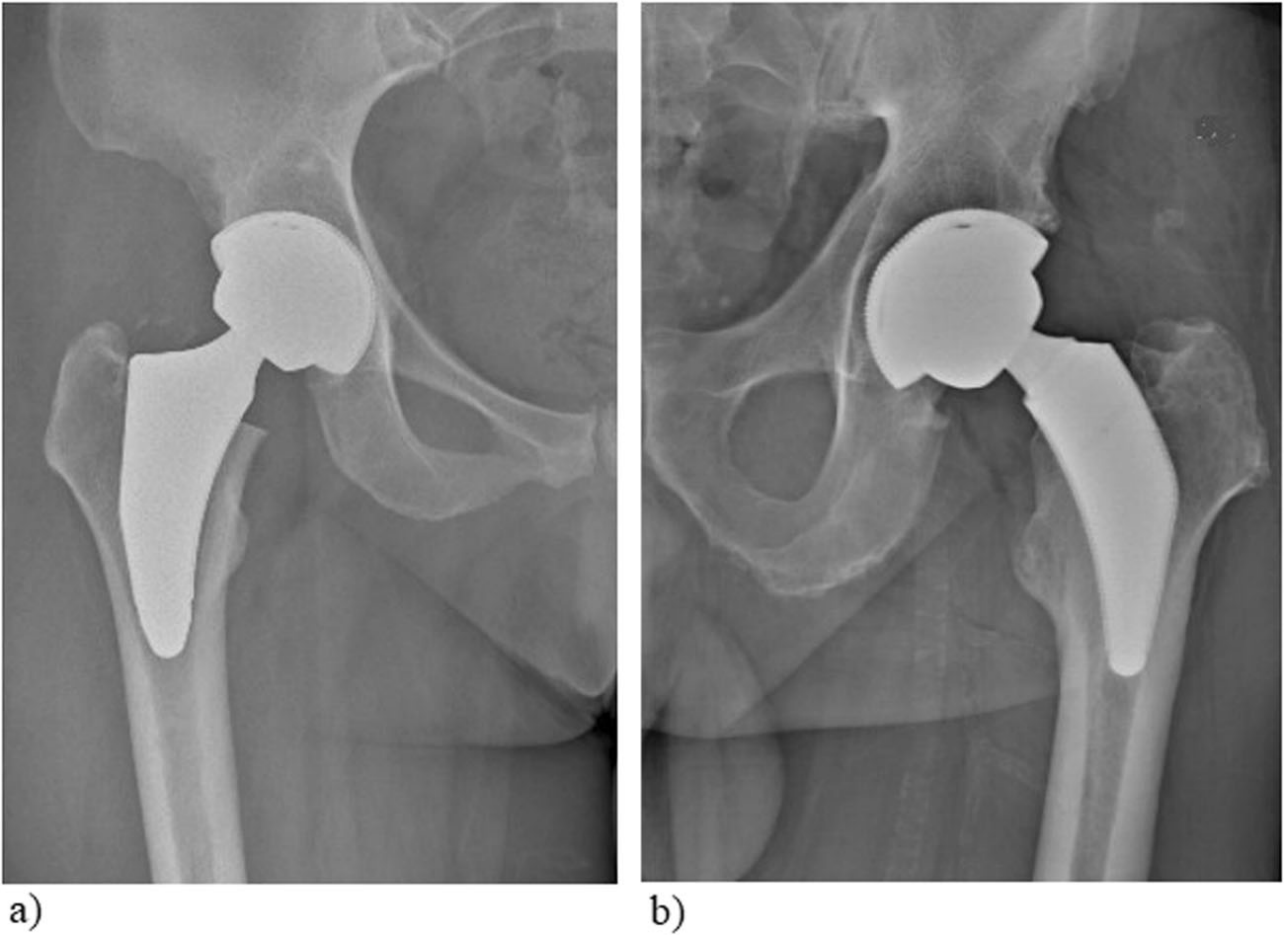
Radiographic images of the stems 2 years after surgery. a Pulchra, Adler Ortho, Milan Italy, b Parva, Adler Ortho, Milan Italy
